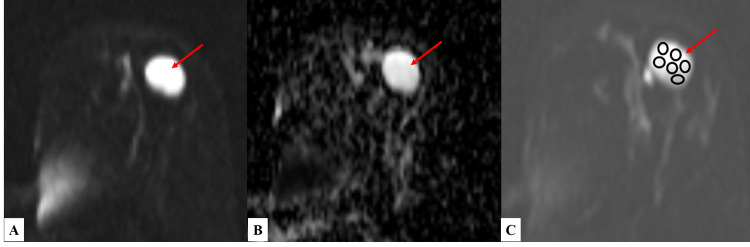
DWI and ADC sequences of a patient with fibroadenoma (A) DWI and (B) corresponding ADC images showing no restriction of diffusion within the lesion present in the left breast – suggestive of benign etiology. (C) DWI image demonstrating the method of placing multiple ovoid ROIs (each measuring ~25 mm^2^) throughout the benign lesion for calculation of mean ADC value. DWI - diffusion-weighted imaging; ADC - apparent diffusion coefficient; ROI - region of interest
